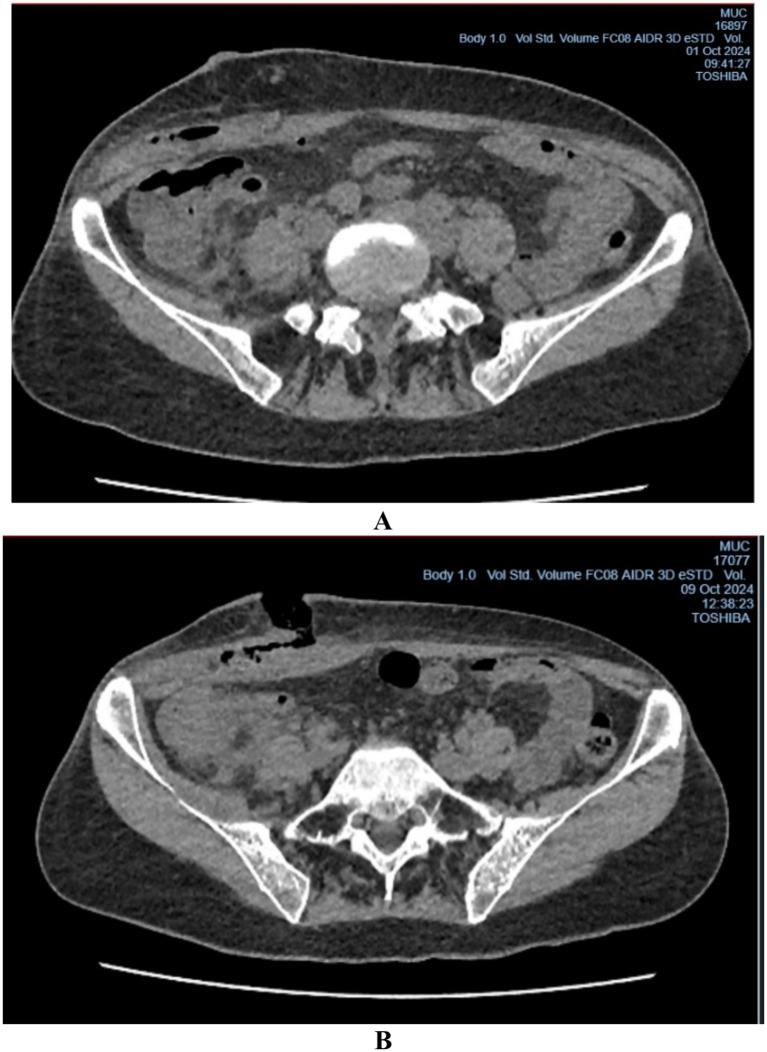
CT image: (A) a subaponeurotic abscess in the area of the postoperative wound in the right iliac region; (B) after the drainage of the subaponeurotic abscess.
